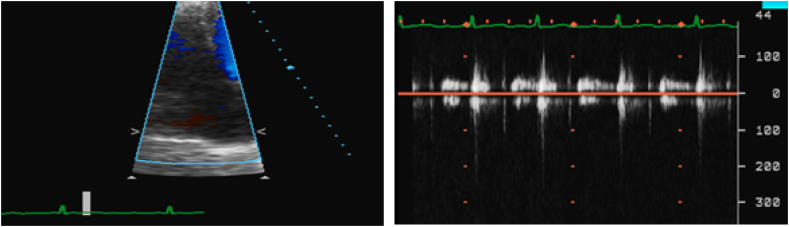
Color & spectral Doppler showing minimal TR. (For interpretation of the references to colour in this figure legend, the reader is referred to the Web version of this article.)
